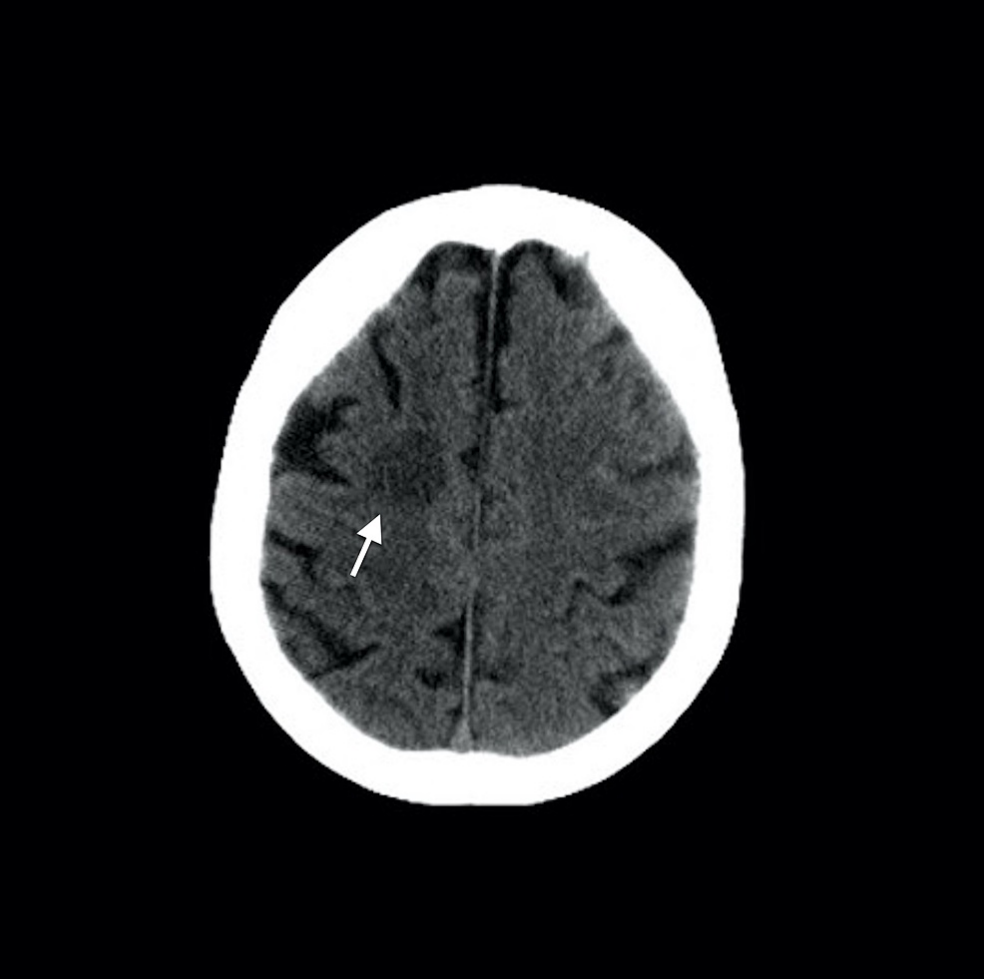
Hypodense nodular lesion with edema in right frontal region is present in non-contrast enhanced computed tomography.
